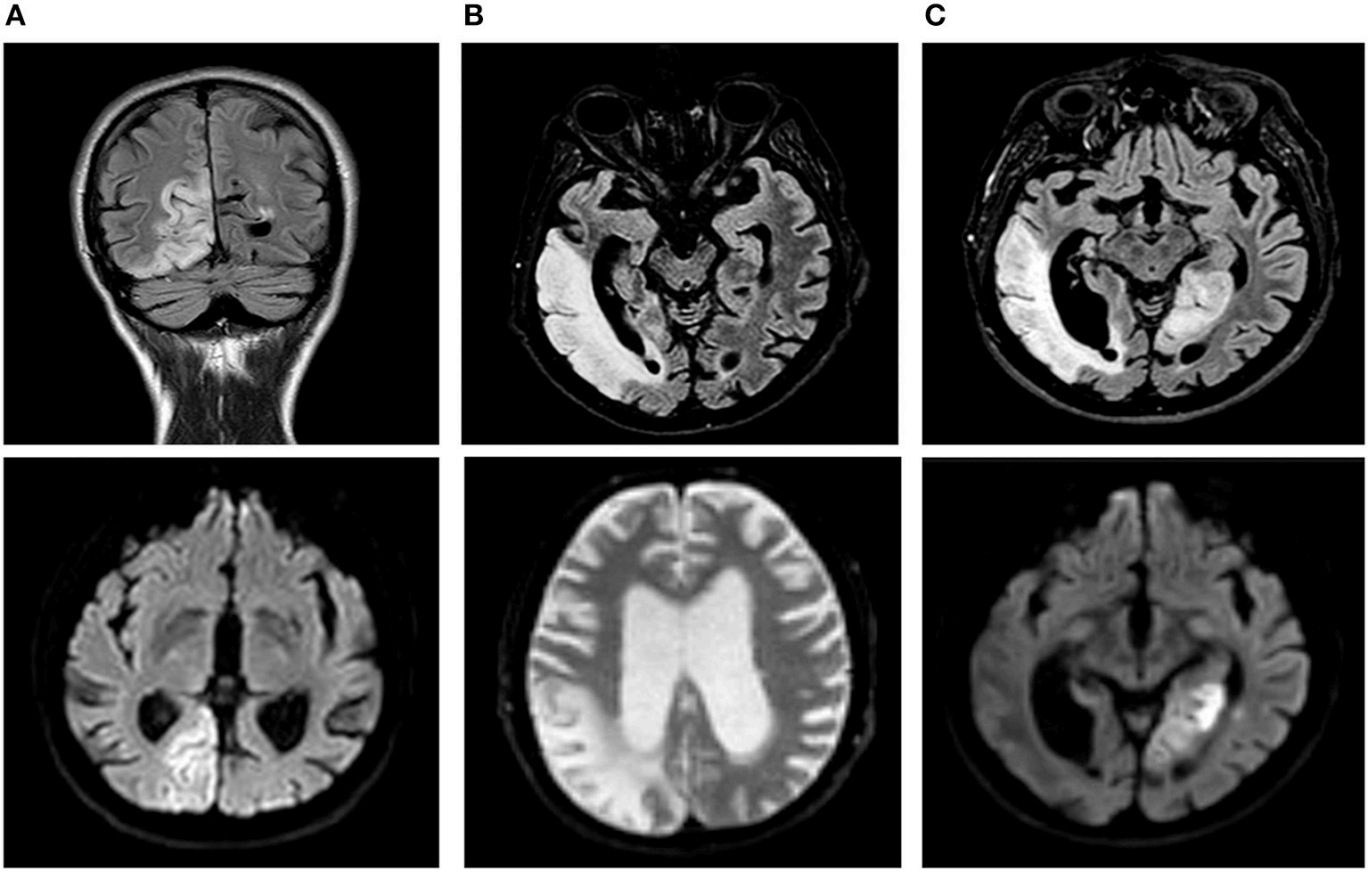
Temporal evolution of brain magnetic resonance imaging (MRI). FLAIR (fluid attenuated recovery) sequences are shown in the upper line, and DWI (diffusion-weighted imaging) sequences are shown in the lower line. (A) First stroke-like episode initially interpreted as an ischemic stroke. A high signal occurred in the diffusion-weighted imaging (DWI) sequences in the right temporo-occipital lobes and the corresponding fluid attenuated recovery (FLAIR) sequence. (B) Second stroke-like episode: three months later, evidence of a wider lesion was found in the right temporal, parietal and occipital lobes together with initial enlargement of the ventricular spaces. (C) Third stroke-like episode: a new cortical DWI abnormality was observed in the left medial temporal and occipital lobes; marked and diffuse atrophy was found in the brain parenchyma.
